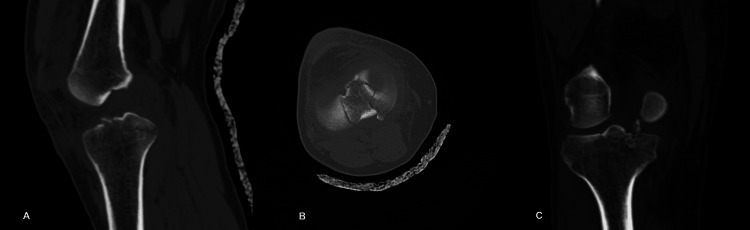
Patient's right knee CT. (A) CT sagittal view. (B) CT transverse view. (C) CT coronal view. CT: computed tomography
